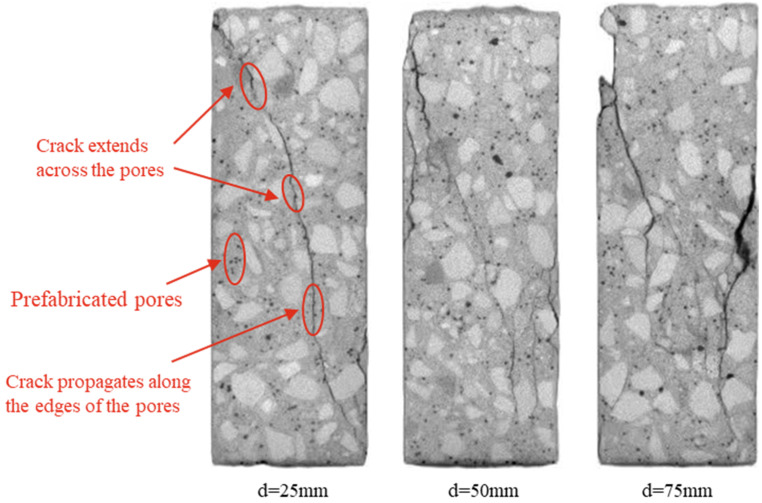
CT images of longitudinal sections at $P_{\mathrm{pore}}=8\%$ with pore size of 2–3 mm.
